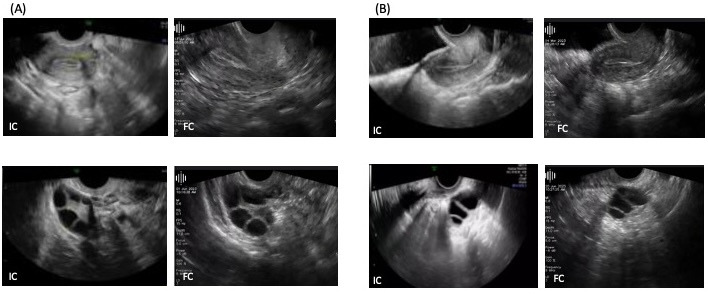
IC and FC scans taken from 2 representative patients on the same day ((A) patient A and (B) patient B). FC: Pulsenmore follicle count home vaginal ultrasound; IC: in-clinic.
